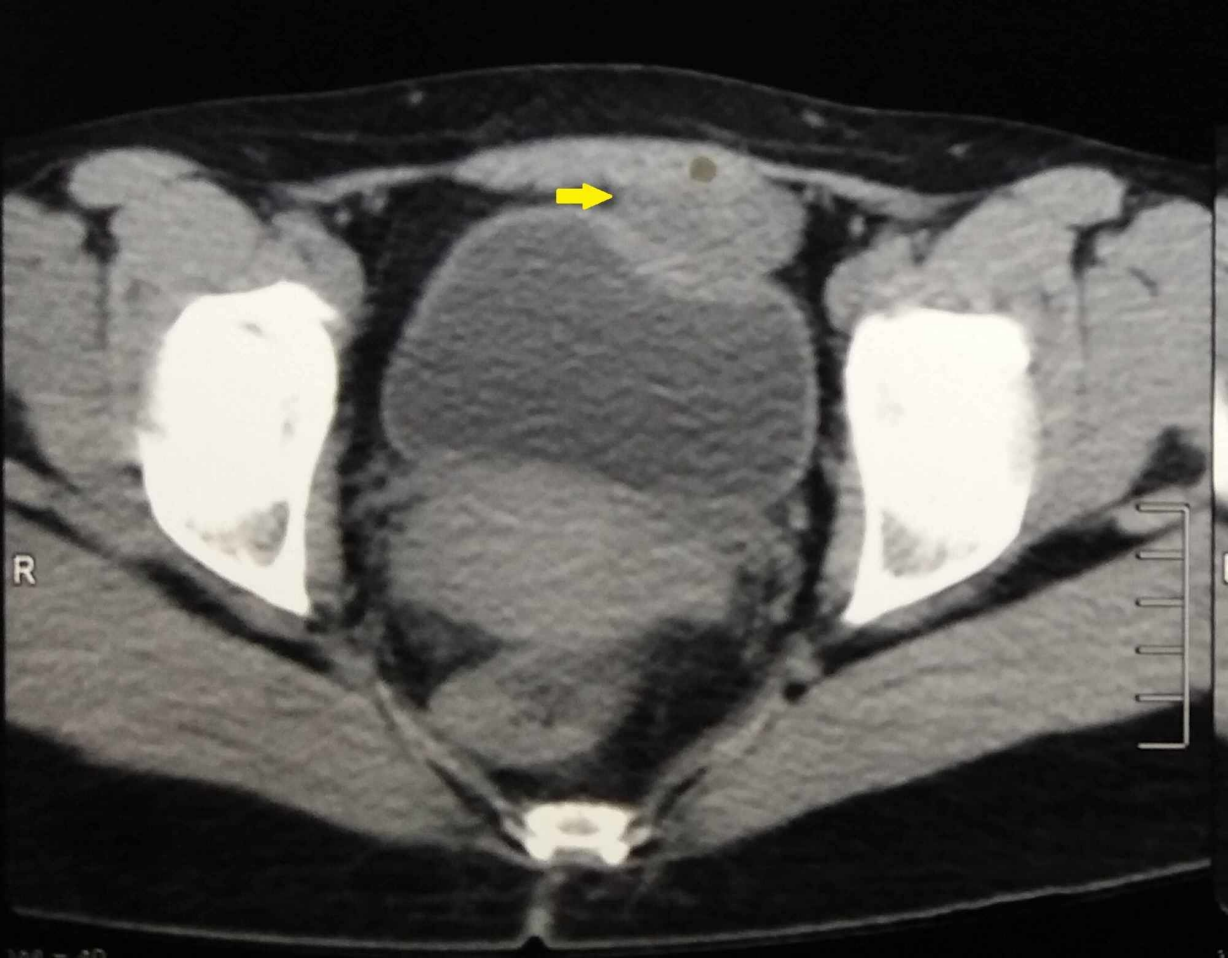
Transverse section of the CT showing the urachal cyst (arrow) anterior to the bladder CT: computed tomography
